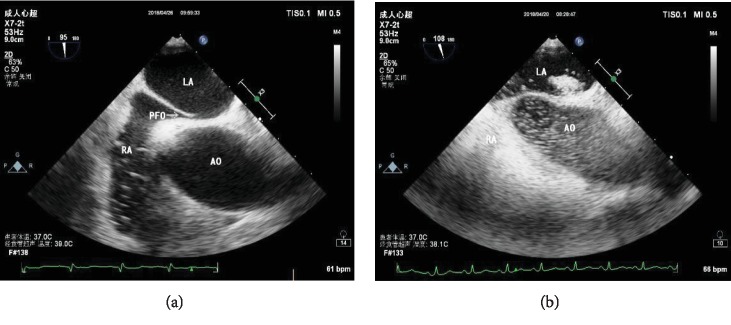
Transesophageal echocardiography showing (a) the morphology of PFO and (b) contrast agent passing through the PFO. LA: left atrium; RA: right atrium; AO: aorta.
